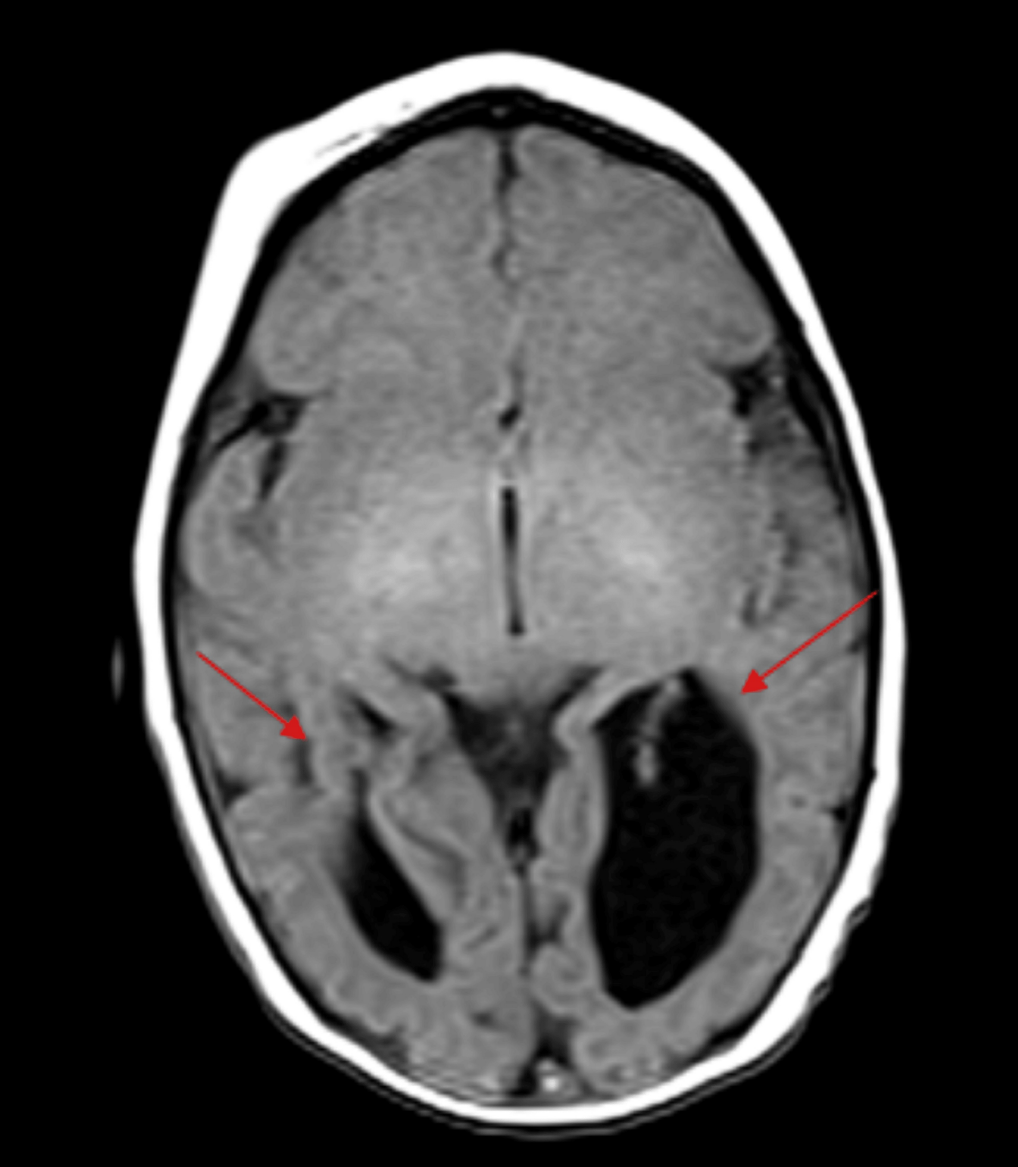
T1 axial view brain MRI showing bilateral cerebral dysgenesis at the occipital head region (arrows). MRI: magnetic resonance imaging
